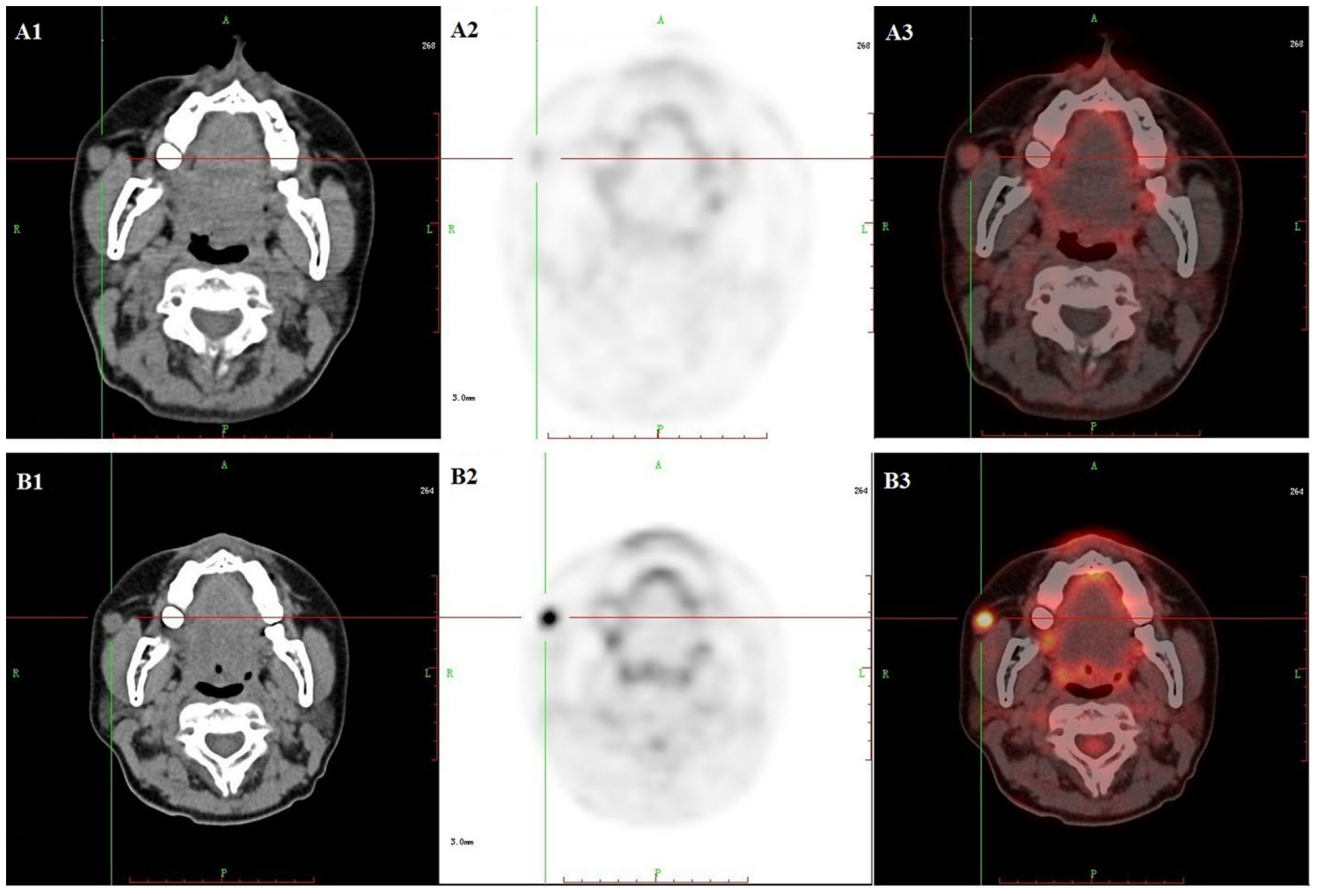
Comparison of different uptake patterns in ^18^F-AIF-NOTA-PRGD2 PET and ^18^F-FDG PET. The enlarged lymph node identified on CT (A1 and B1) had significantly higher uptake of ^18^F-FDG PET (B2–B3) than that for ^18^F-AIF-NOTA-PRGD2 (A2–A3).
